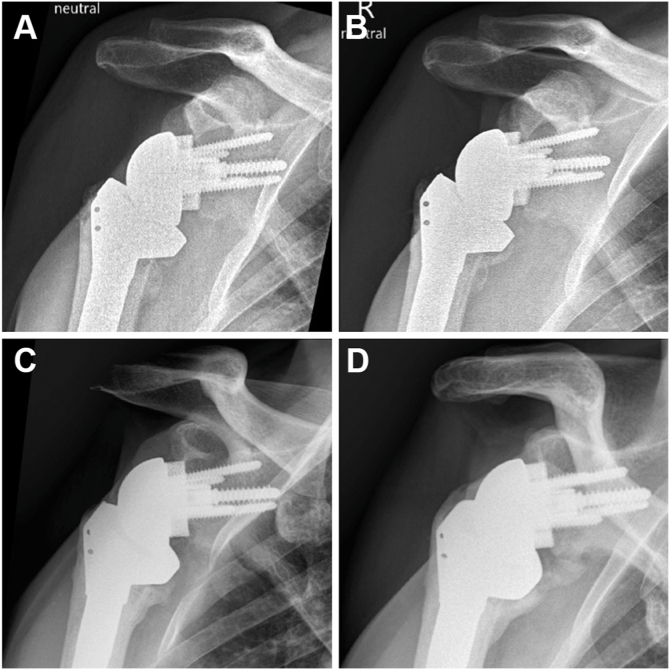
Progressive glenohumeral heterotopic ossification seen on anteroposterior radiographs of the right shoulder at (A) 6 weeks, (B) 6 months, (C) 1 year, and (D) 2 years after reverse arthroplasty.
